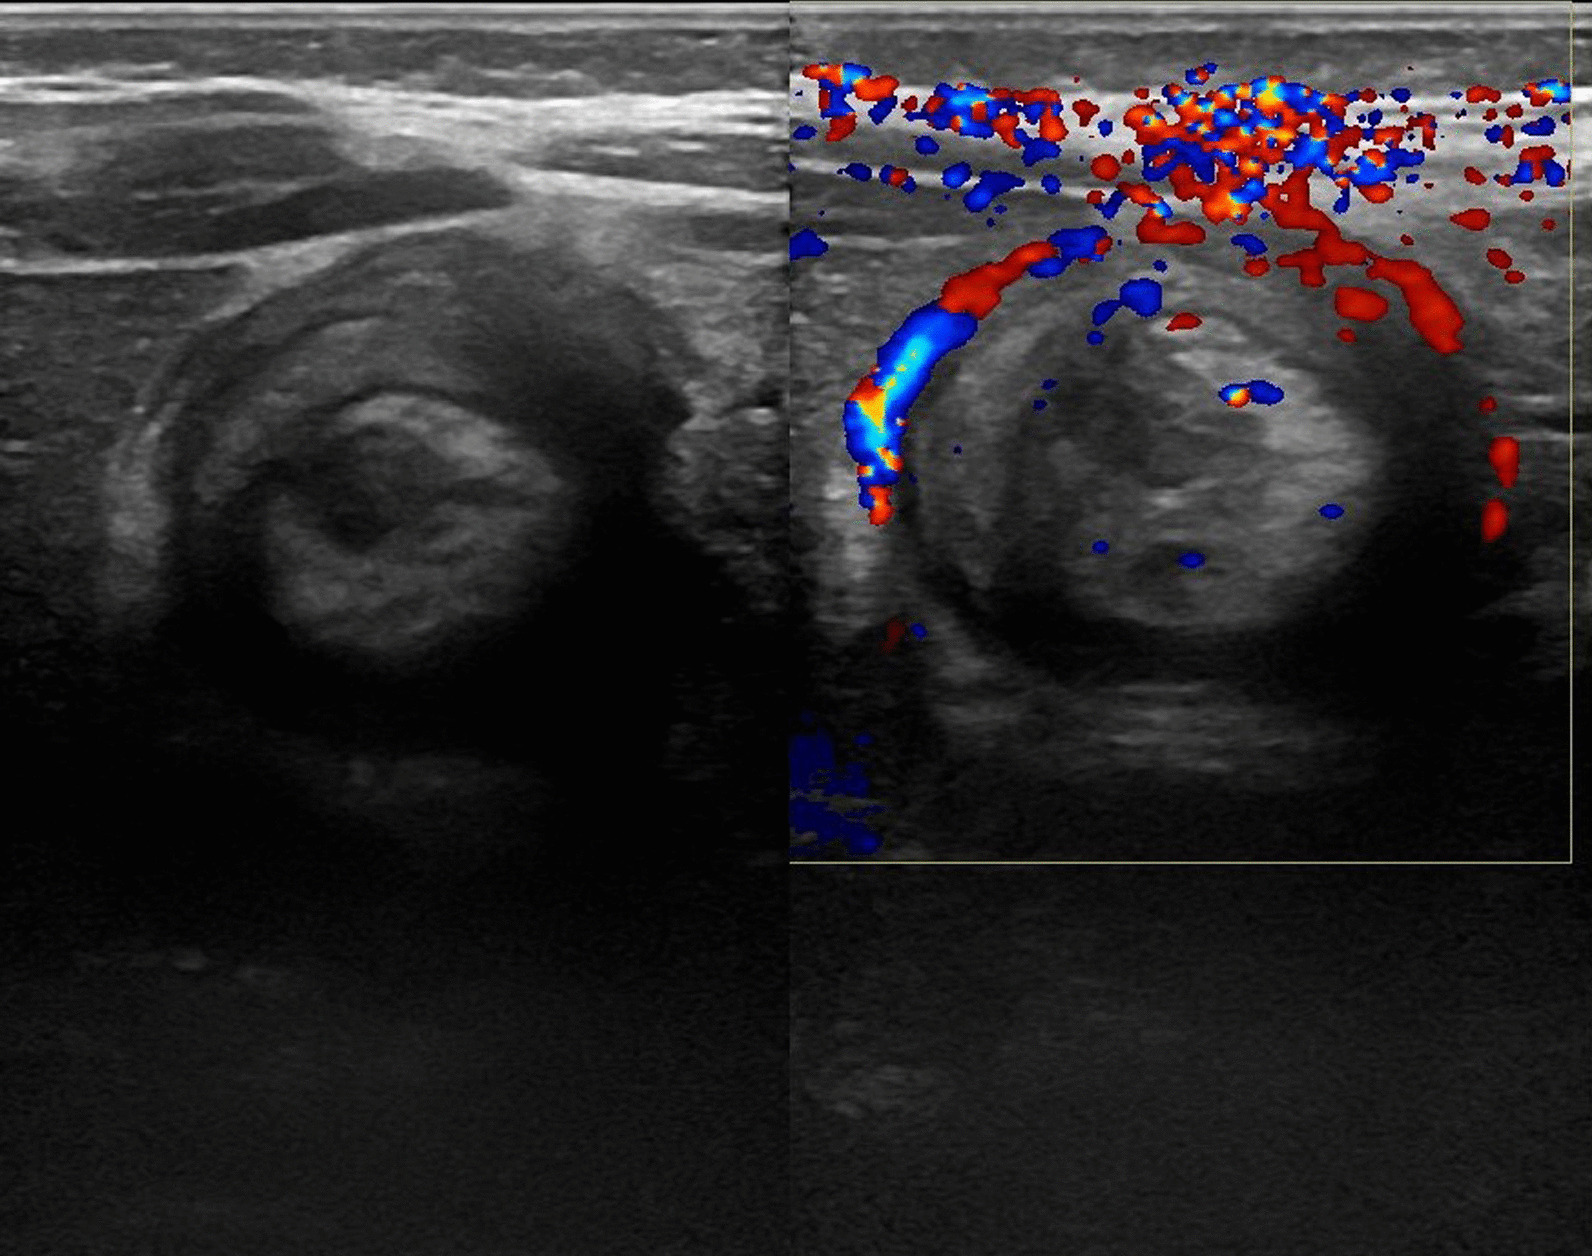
Ultrasound showing target sign with peripheral vascularity
